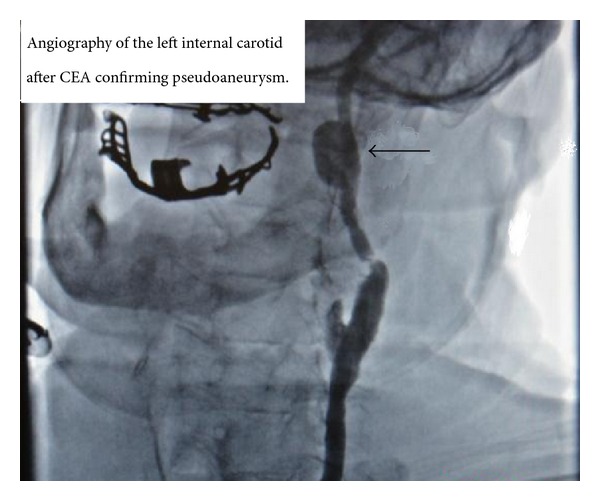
Computed tomography angiography of left internal carotid artery; pseudoaneurysm location is confirmed.
